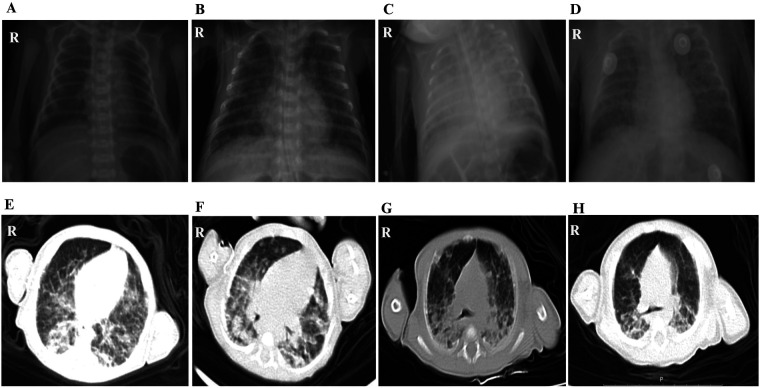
The lung imaging changes of a very premature child of 27^+5^ weeks from birth to diagnosis of CTB to 70 days after treatment. (A) Chest radiograph at birth. (B) Chest radiograph of CTB was confirmed on the 29th day after birth. (C) Chest radiograph on the 38th day after birth, the 9th day after anti-TB treatment. (D) Chest radiograph on the 45th day after birth, the 16th day after anti-TB treatment. (E) Chest CT scan images on the 57th day after birth, the 28th day after anti-TB treatment. (F) Chest CT scan on the 71st day after birth, the 42nd day after anti-TB treatment. (G) Chest CT scan on the 85th day after birth, the 56th day after anti-TB treatment. (H) Chest CT scan images on the 99th day after birth, the 70th day after anti-TB treatment.
